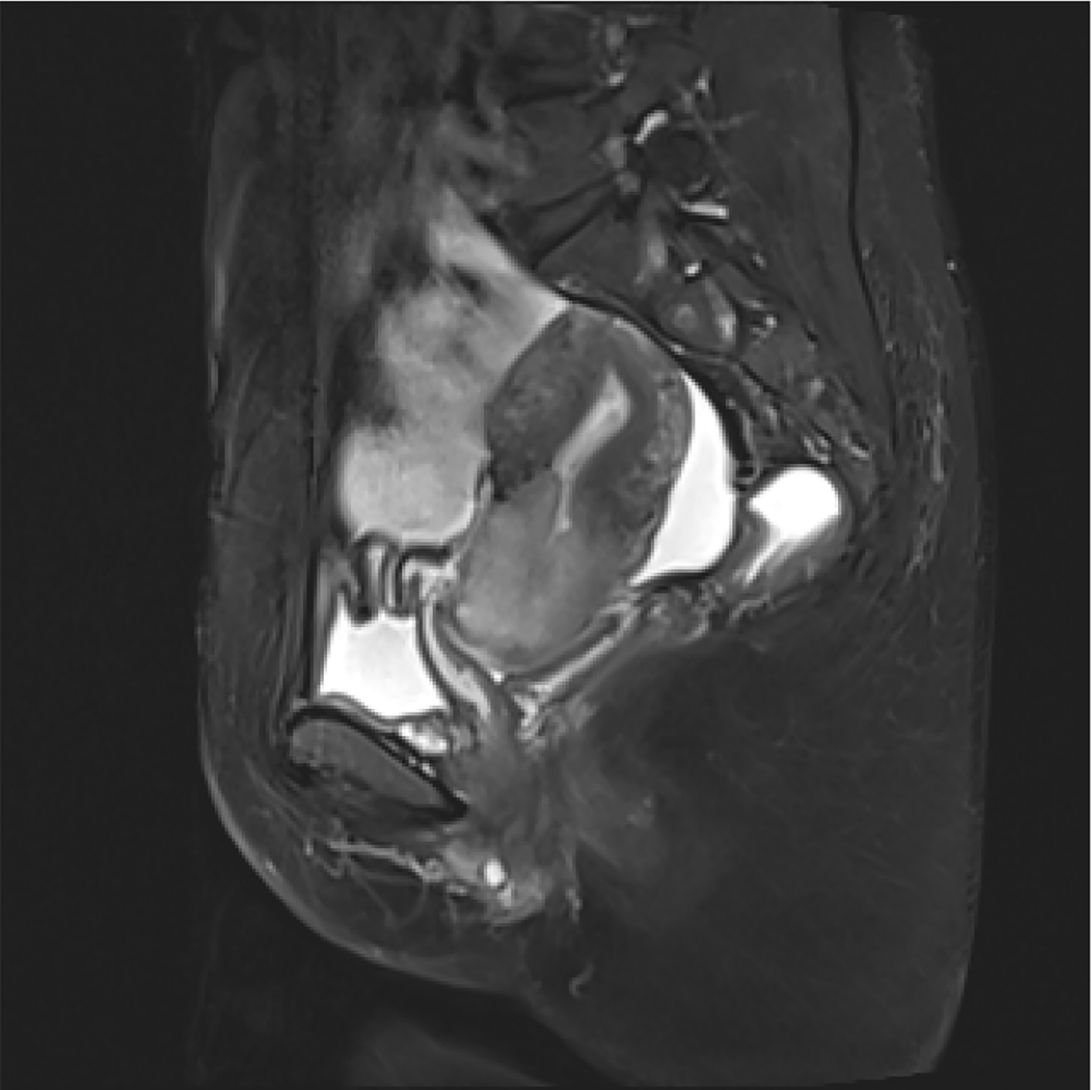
Pelvic magnetic resonance imaging (MRI) showed a cervical tumor 40 mm * 36 mm * 48 mm in size, without invasion of the uterus and vagina, and no pelvic lymph node metastasis.
